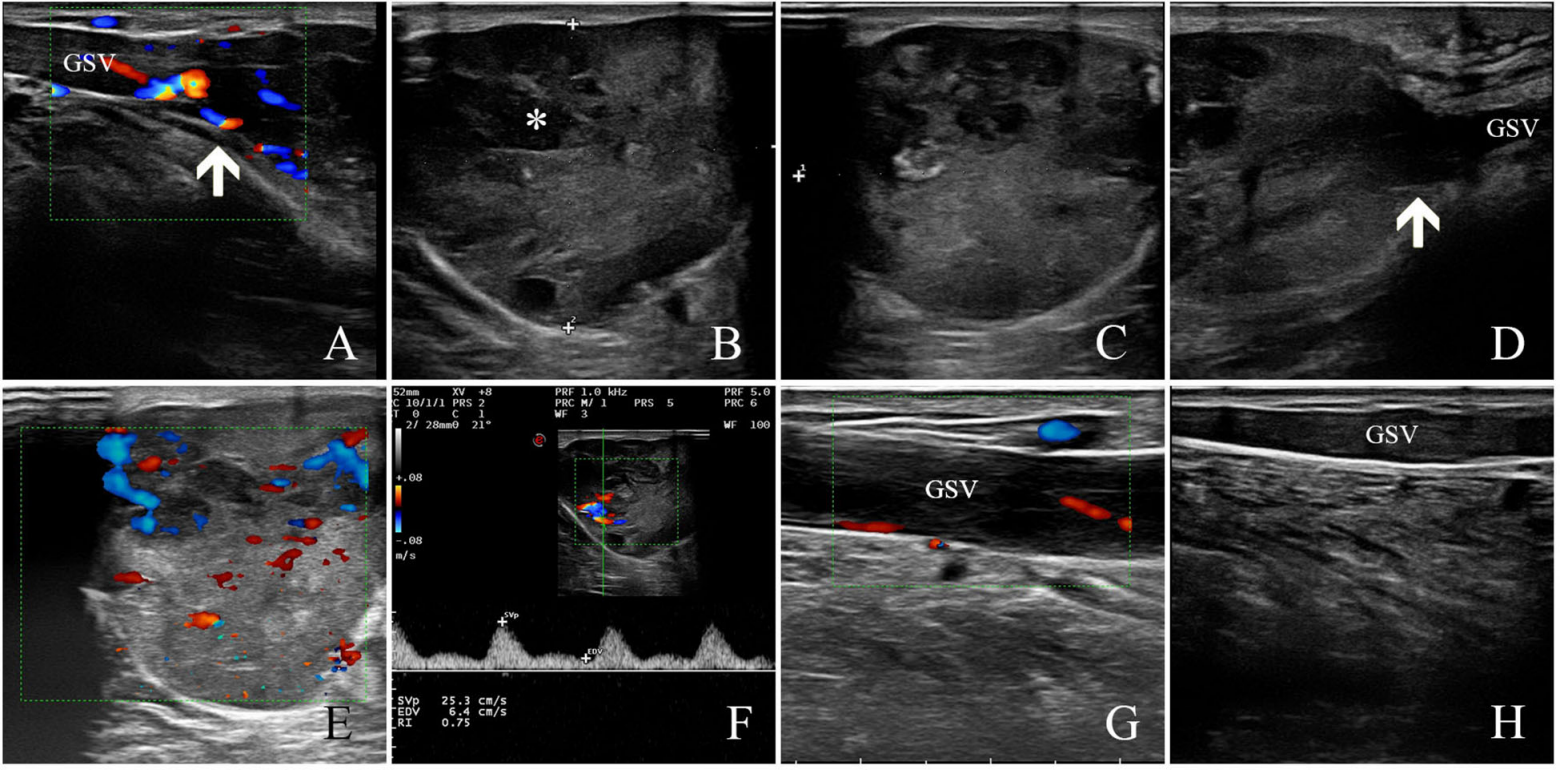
Ultrasound findings of GSV-LMS in a male patient. (A) The white arrow indicates the upper pole of the lesion. (B, C) The figure shows that the internal echo was heterogeneous hypoechoic, and a number of honeycomb-like anechoic areas were detected (as shown by white * in the figure). (D) The white arrow indicates the lower pole of the lesion. (E) Color Doppler flow imaging (CDFI) showed abundant blood flow signals in the mass. (F) The arterial spectrum was detected in the internal blood flow signal of the lesion with a resistance index of approximately 0.75. (G) The GSV at the proximal side of the lesion was full of heterogeneous hypoechoic mass, and a punctured blood flow signal could be detected. (H) The GSV at the distal side of the lesion was full of heterogeneous hypoechoic mass, and no blood flow signal could be detected. GSV, great saphenous vein; LMS, leiomyosarcoma.
